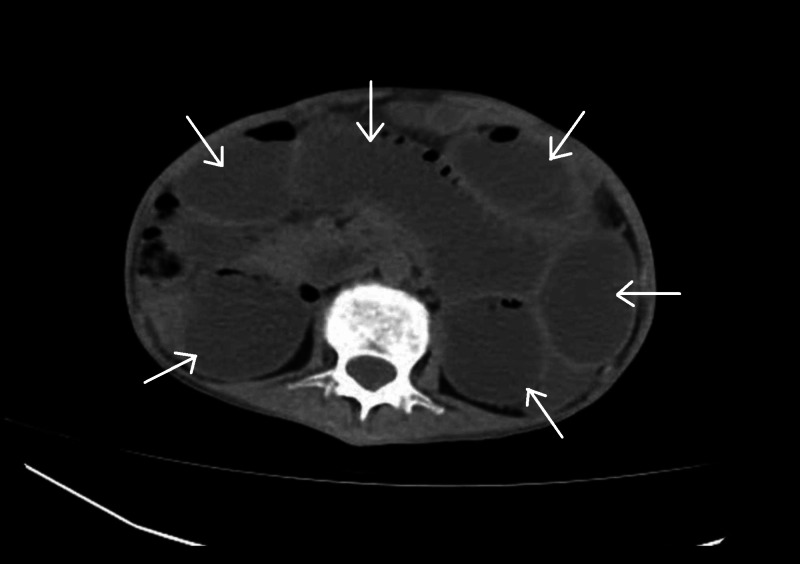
Preoperative axial computed tomography (CT) scan of the abdomen showing dilated small bowel loops (arrows).
